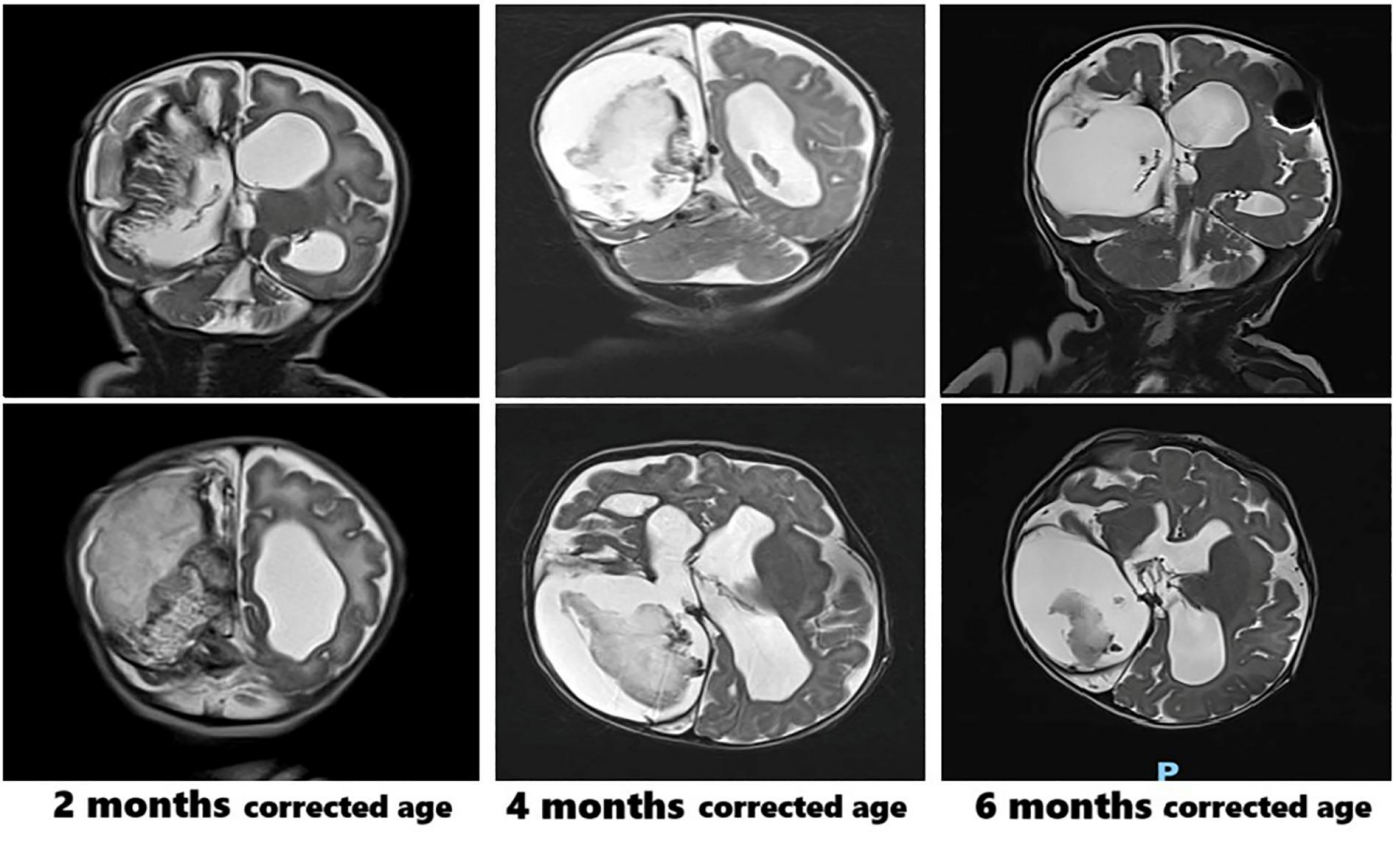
Brain MRI scans at 2, 4, and 6 months corrected age.
